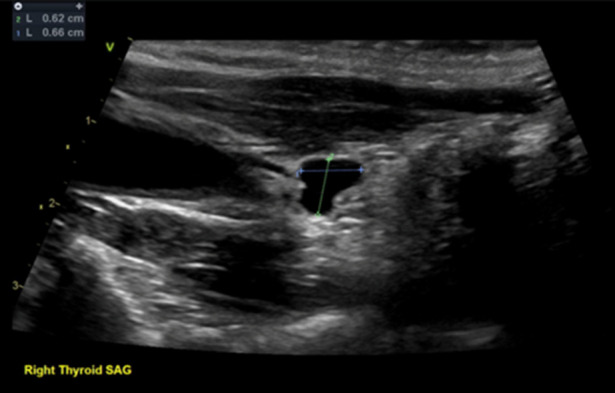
Ultrasound image of patient’s thyroid gland
